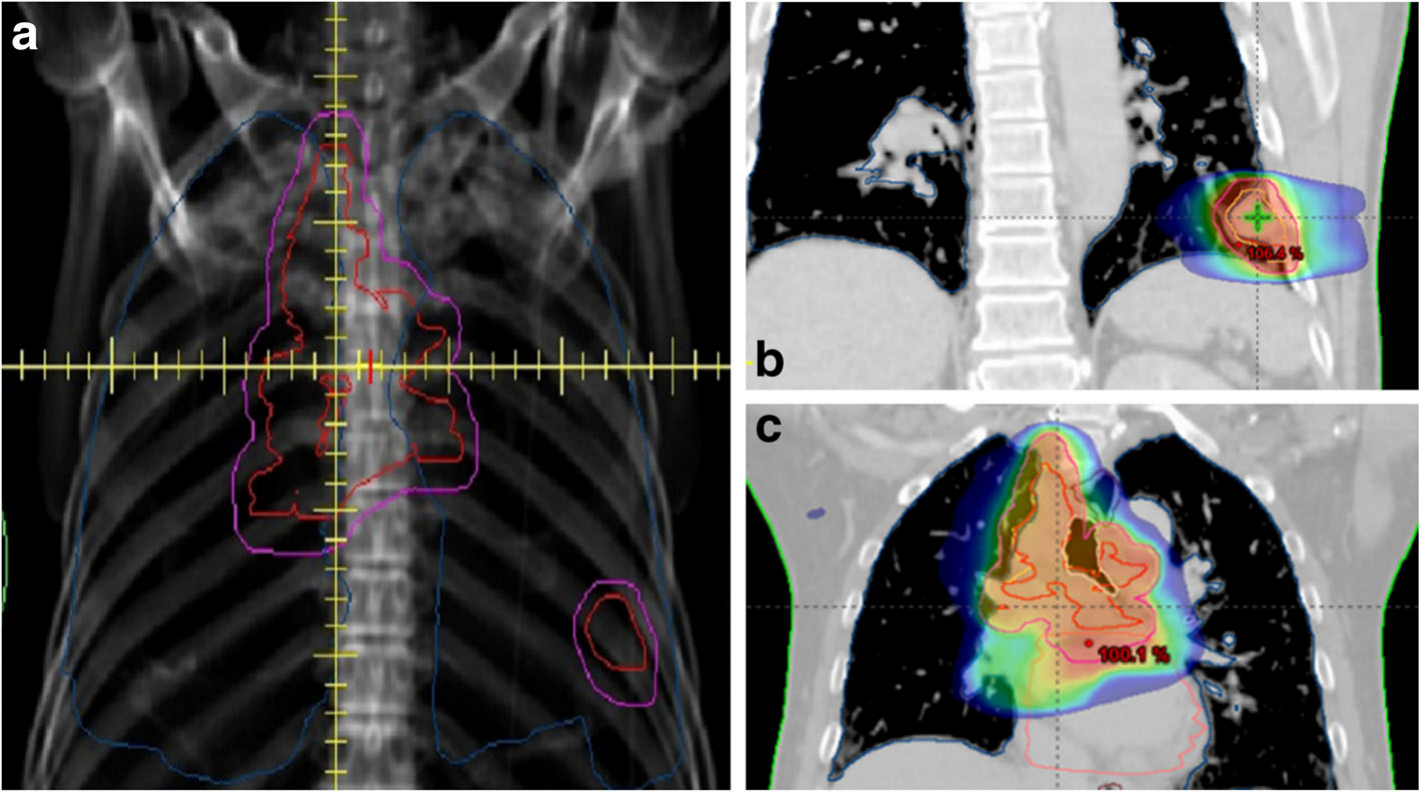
Anterior digitally reconstructed radiography (a) and dose distributions in a 65-year-old man treated with stereotactic body radiotherapy for a 2.4 cm-sized primary tumor in the left lower lobe (b), and with conventional radiotherapy for metastatic mediastinal lymph nodes (c)
